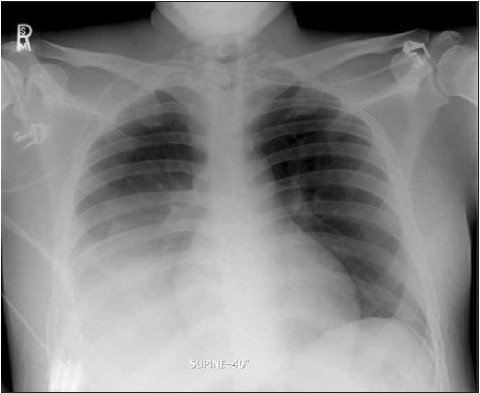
Chest x-ray three weeks after presentation, showing persistent right lower lobe consolidation.
